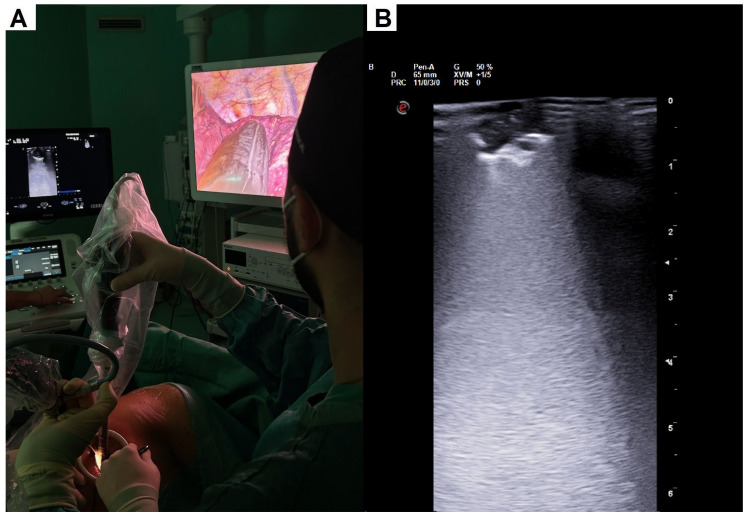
(A) Nodule identification with intra-operative lung ultrasound in uniportal VATS. Instrumental disposition from top to bottom—camera, ILU probe, and retraction forceps. (B) Ultrasound visualization of a lung nodule, with irregularity of the pleural line and posterior hyperechoic reinforcement.
